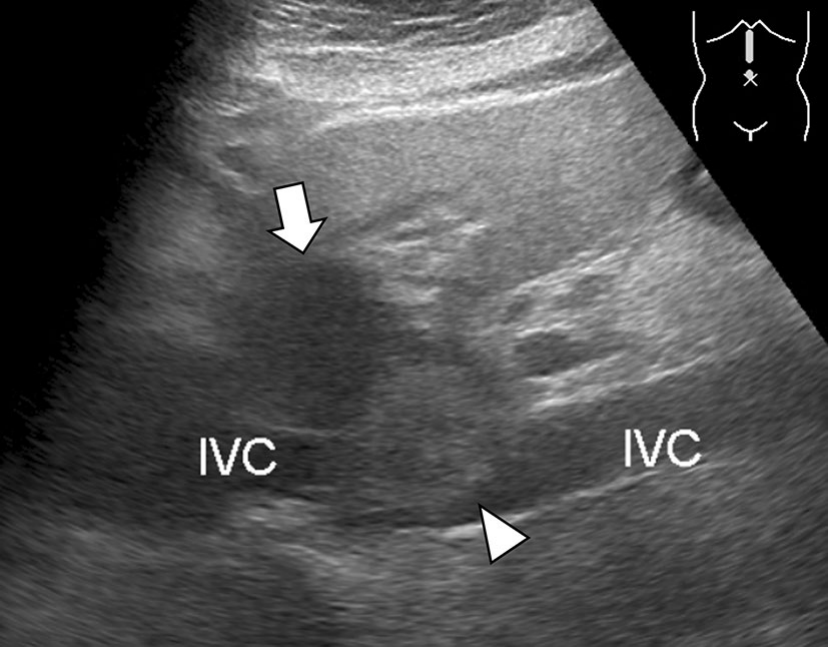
Abdominal ultrasound reveals that the tumor is composed of two distinct components on the ventral and dorsal sides in S1 of liver. The ventral portion shows low echogenicity (arrow) and the dorsal portion shows high echogenicity (arrowhead). The latter component touched widely with the inferior vena cava (IVC), and their boundary is clear
